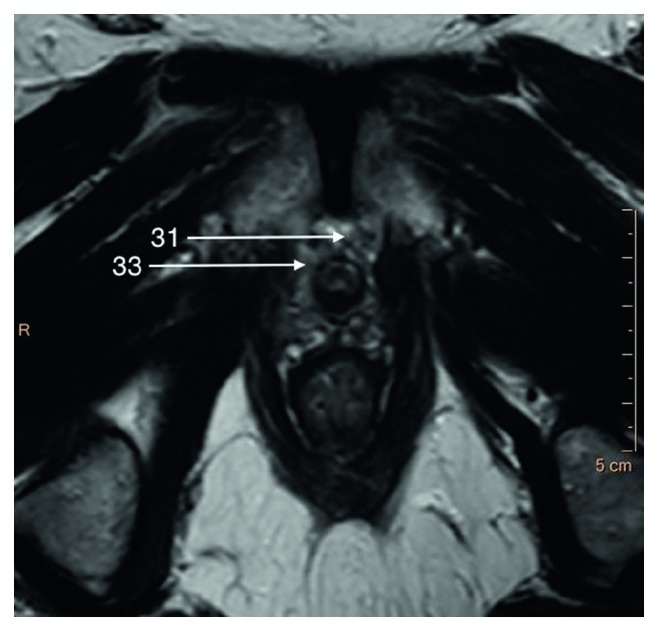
T2-weighted axial image of an apical part of the prostate gland.
